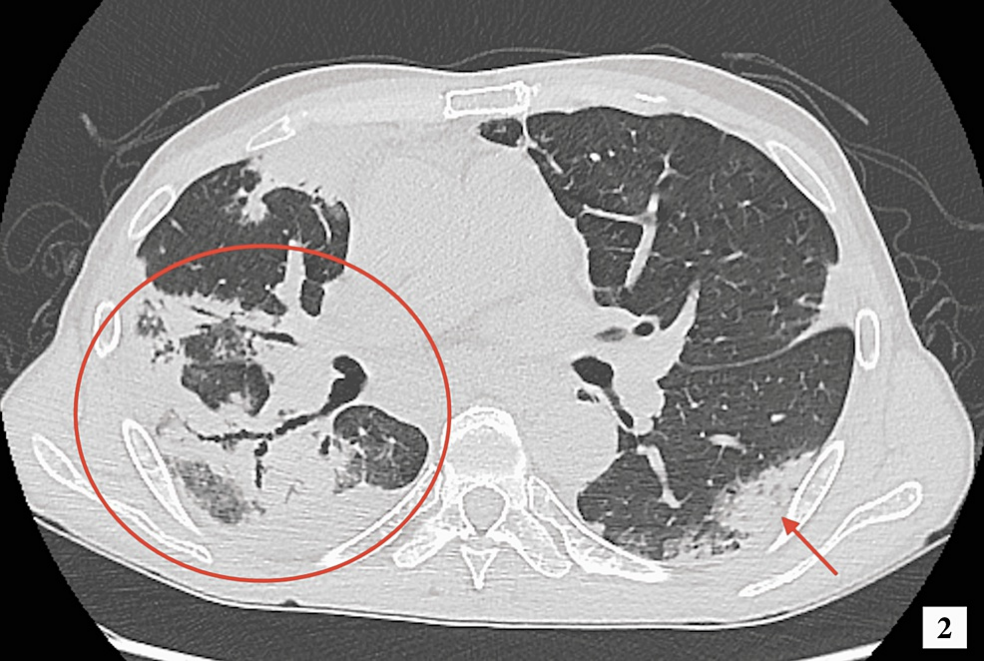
Computed tomography scan of the thorax showing extensive consolidation and ground-glass opacities with traction bronchiectasis.
